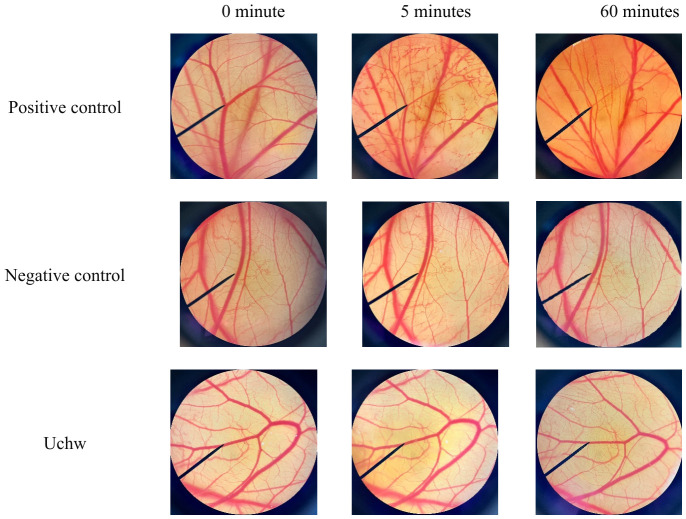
HET-CAM response to positive control (1% *w*/*v* sodium lauryl sulfate) and negative control (0.9% *w*/*v* normal saline solution) at various times of exposure; Uchw = ultrasound-assisted extraction with water.
